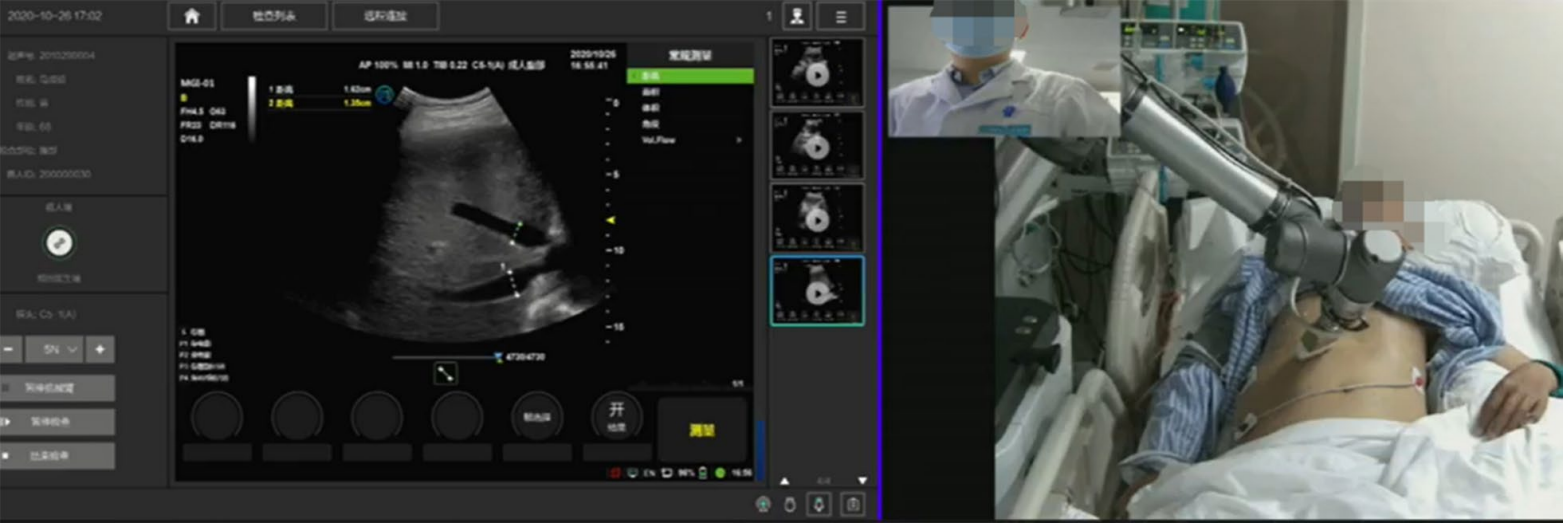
This is a real-time picture of robot-assisted teleultrasound diagnosis, which displays clearly the real-time transmitted ultrasonic images, the position of the probe on the patient and the patient's status
